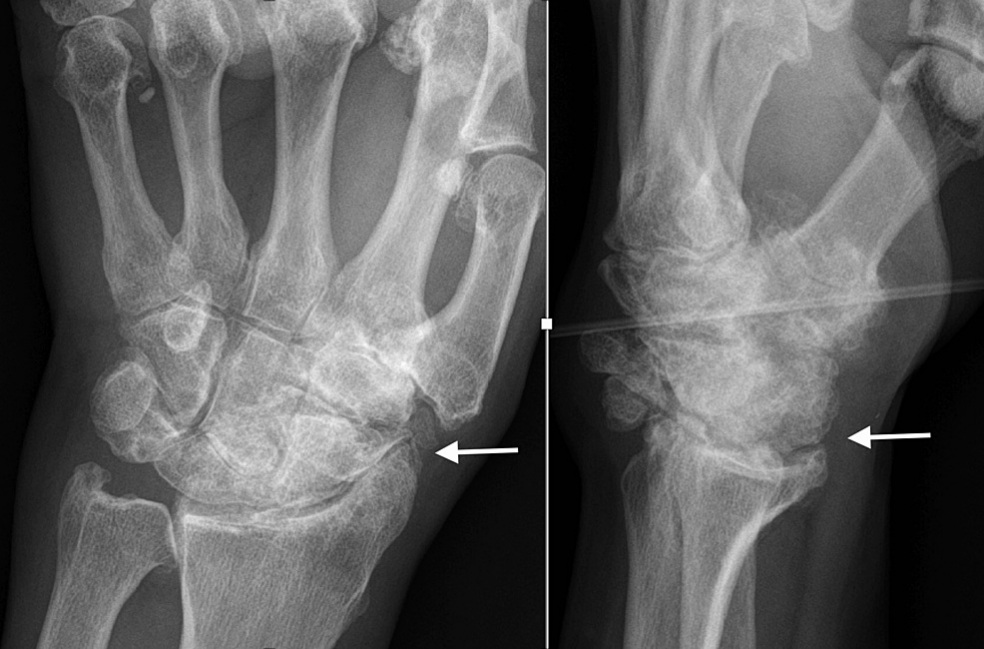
Preoperative radiographs of Case 3 A 73-year-old male with chronic advanced wrist arthritis. The white arrows show the radiocarpal and midcarpal arthritis.
